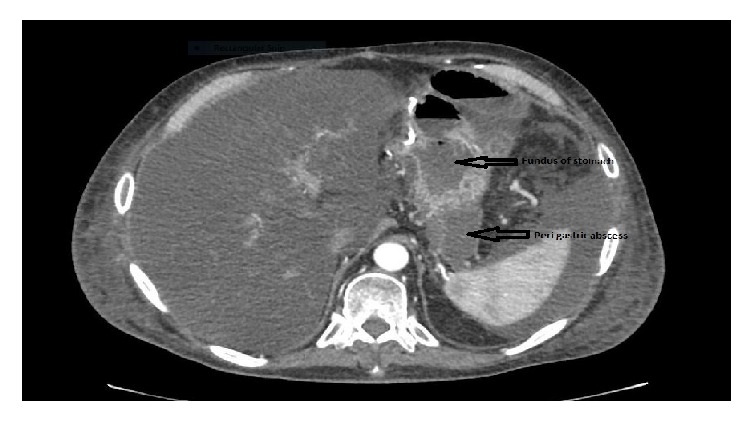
Patient 1, CT abdomen showing perigastric abscess.
